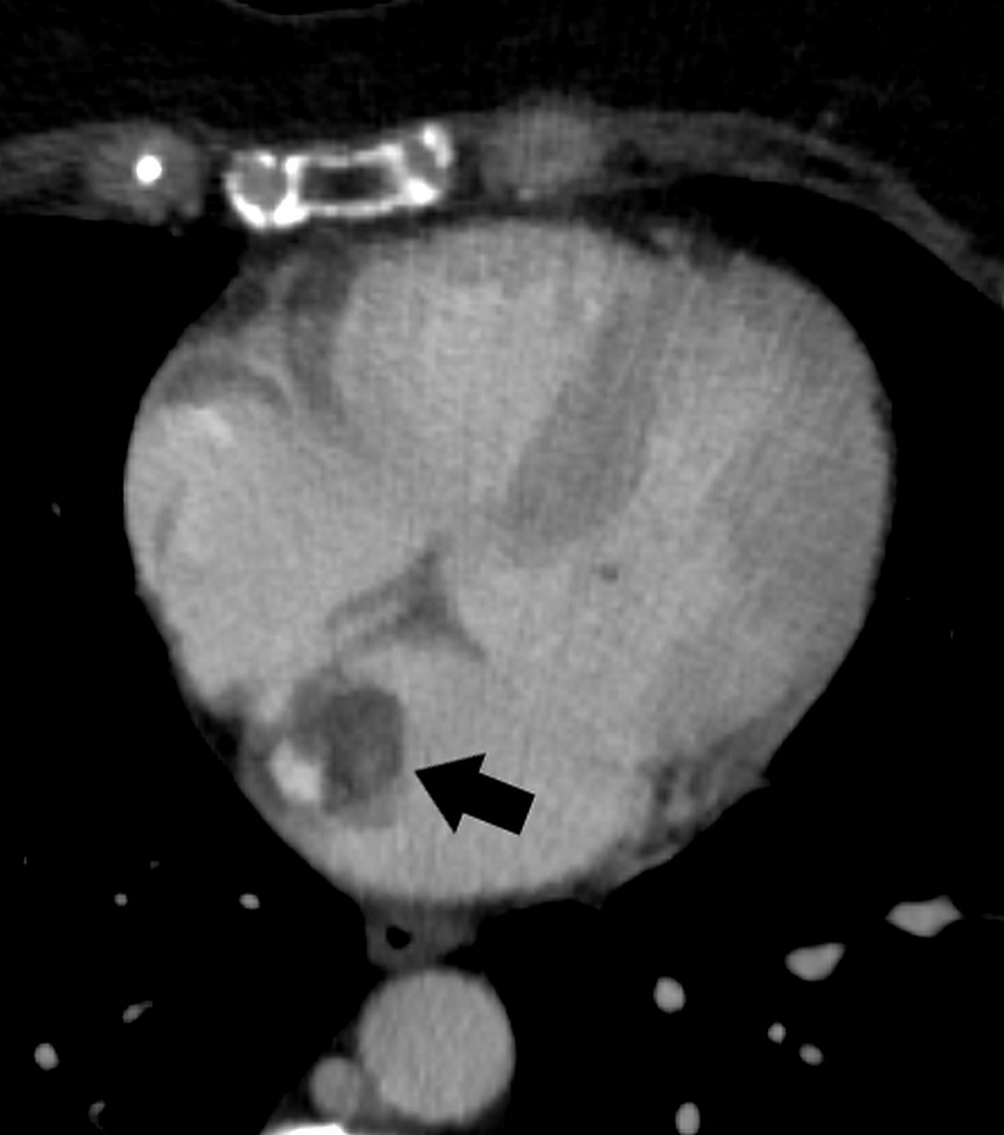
Incidentally detected left atrial myxoma (arrow) on a chest CT
